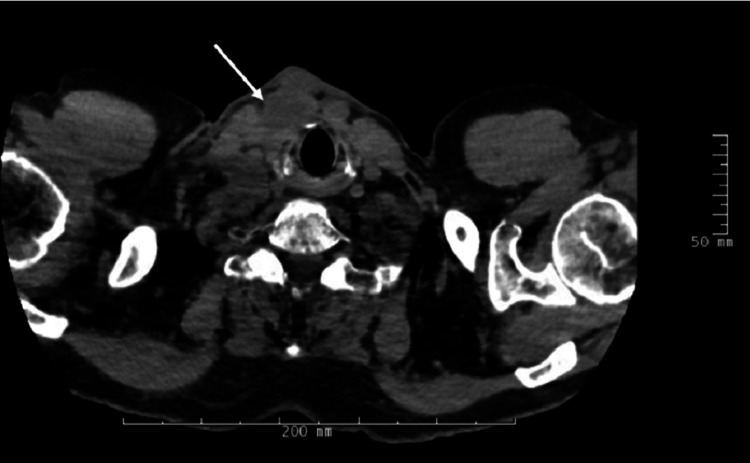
Chest CT without IV contrast
